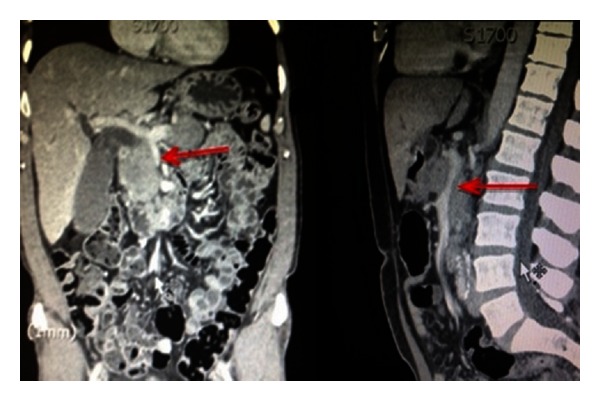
Portal vein narrowed with evidence of impingement on CT axial and sagittal images.
